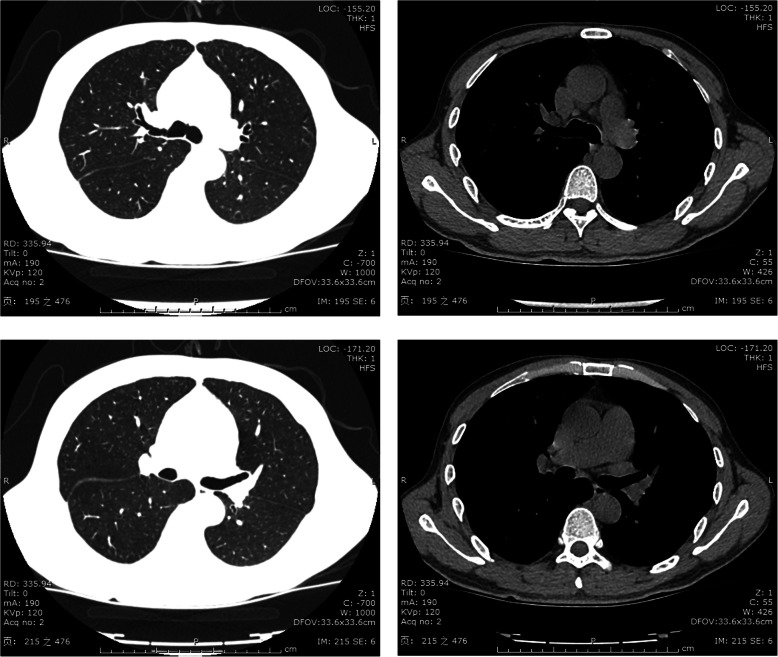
Chest computed tomographic scans of our patient with antineutrophil cytoplasmic antibody–positive infective endocarditis
